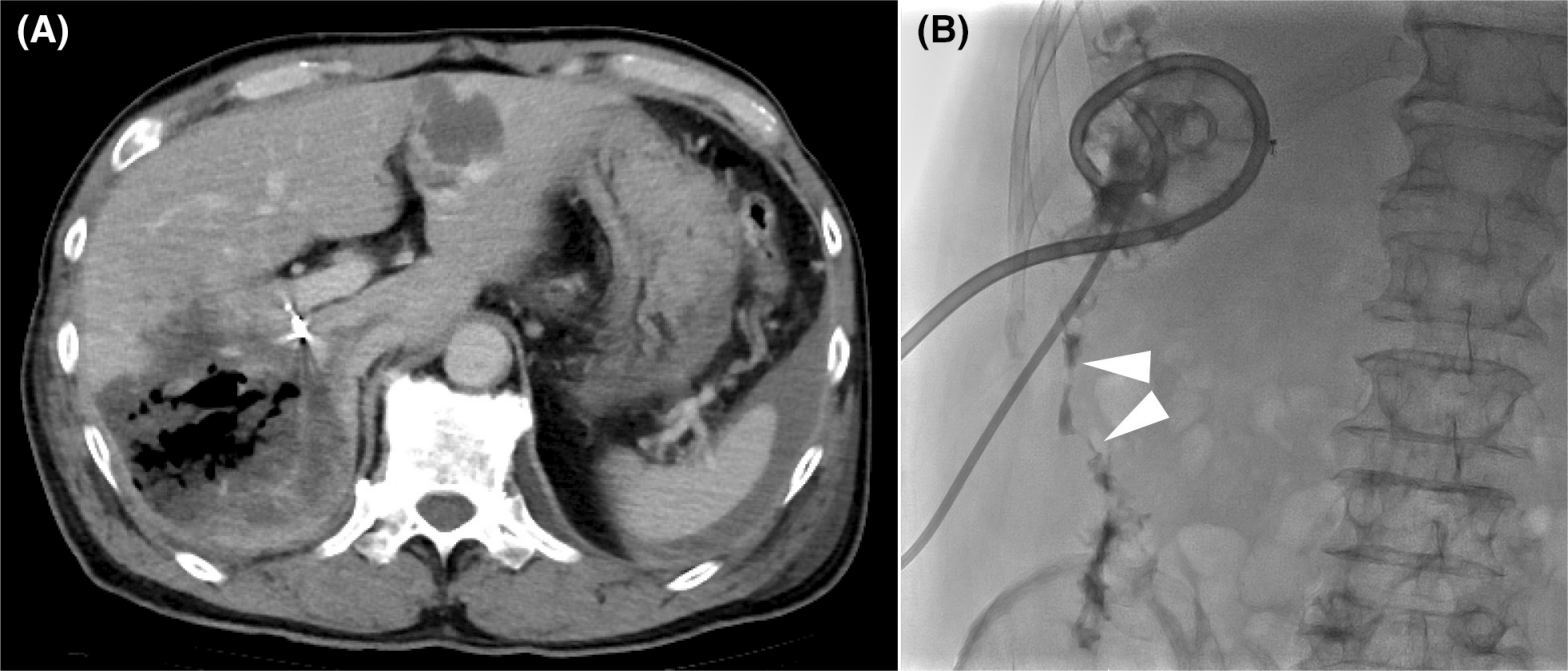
Images following the hemostatic procedure. (A) Computed tomography on the fifth hospital day. There was gas concentrated in the necrotic area of the liver, and infection originating from the bile duct was suspected. Note the presence of a hemostatic coil in the main trunk of the posterior arterial section. (B) Necrotic cavity imaging after placement of two drainage tubes in the area of necrosis. Fistula formation (arrowheads) from the necrotic area to the colon was proven.
